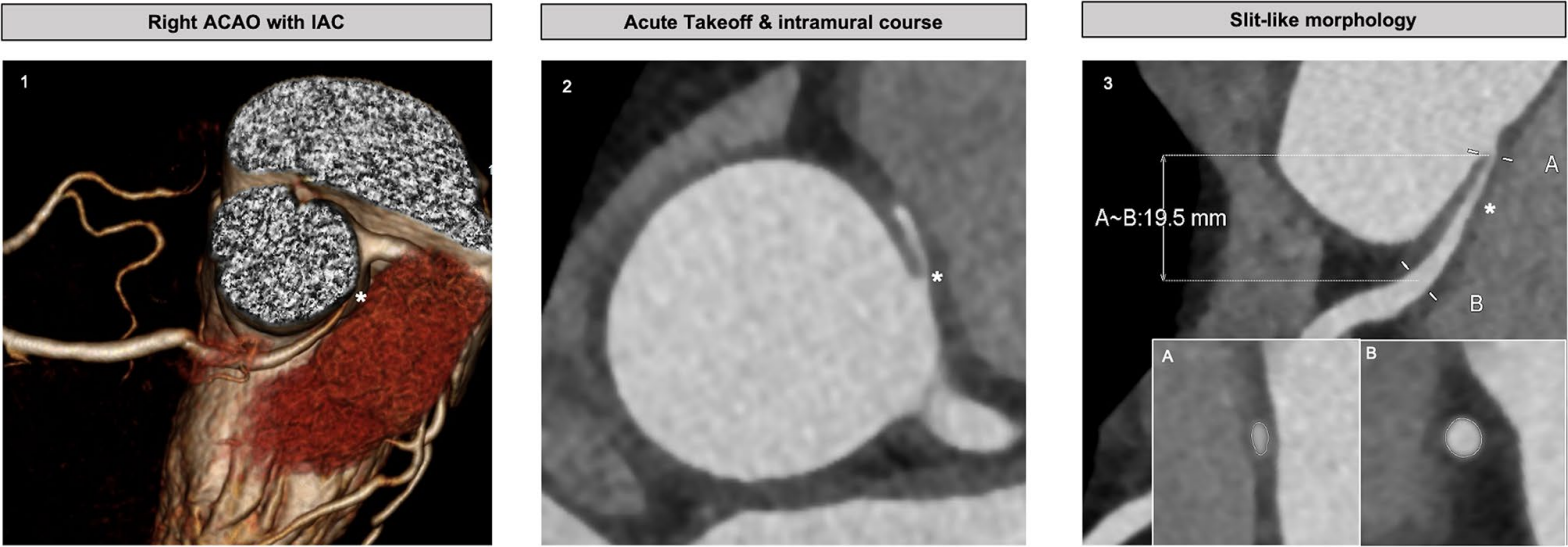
High risk anatomic features of right-ACAOS with IAC on CCTA—CCTA-identified right-ACAOS features. (1) 3D image depicting anomalous origin of the coronary artery arising from the opposite sinus with interarterial course between aorta and pulmonary artery. (2) Intramural location and take-off angles obtained in multiplanar axial reconstructions at the level of the ACAOS ostium. (3) Slit-dlike morphology. Lumen diameters obtained in double oblique view, taking the maximum and minimum diameters of the vessel at the most narrowed proximal location (A) and the distal reference (B). A–B distance represents the interarterial course length of the anomalous vessel. *ACAOS* anomalous origin of the coronary artery arising from the opposite sinus; *CCTA* coronary computed tomography angiography; *IAC* interarterial course.
